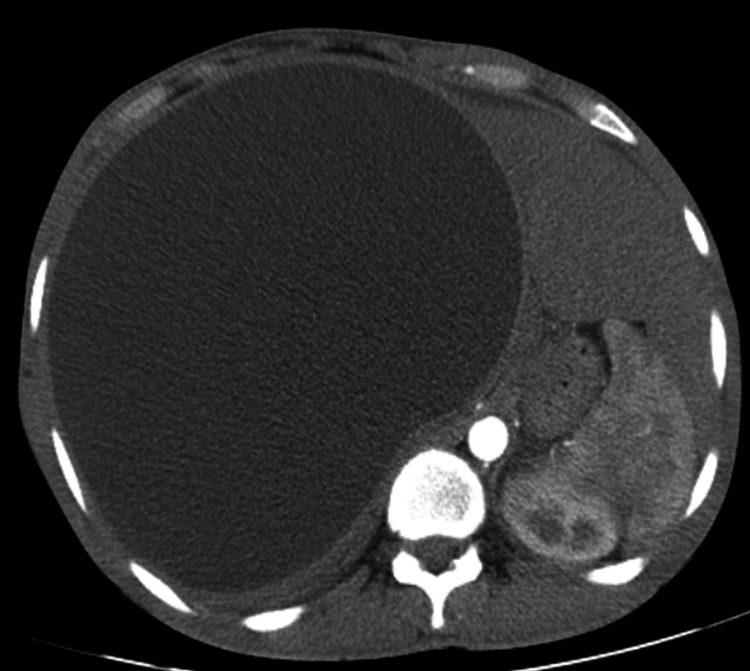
Axial CT scan of the abdomen showing a gigantic liver cyst displacing intra-abdominal organs. CT: computed tomography.
